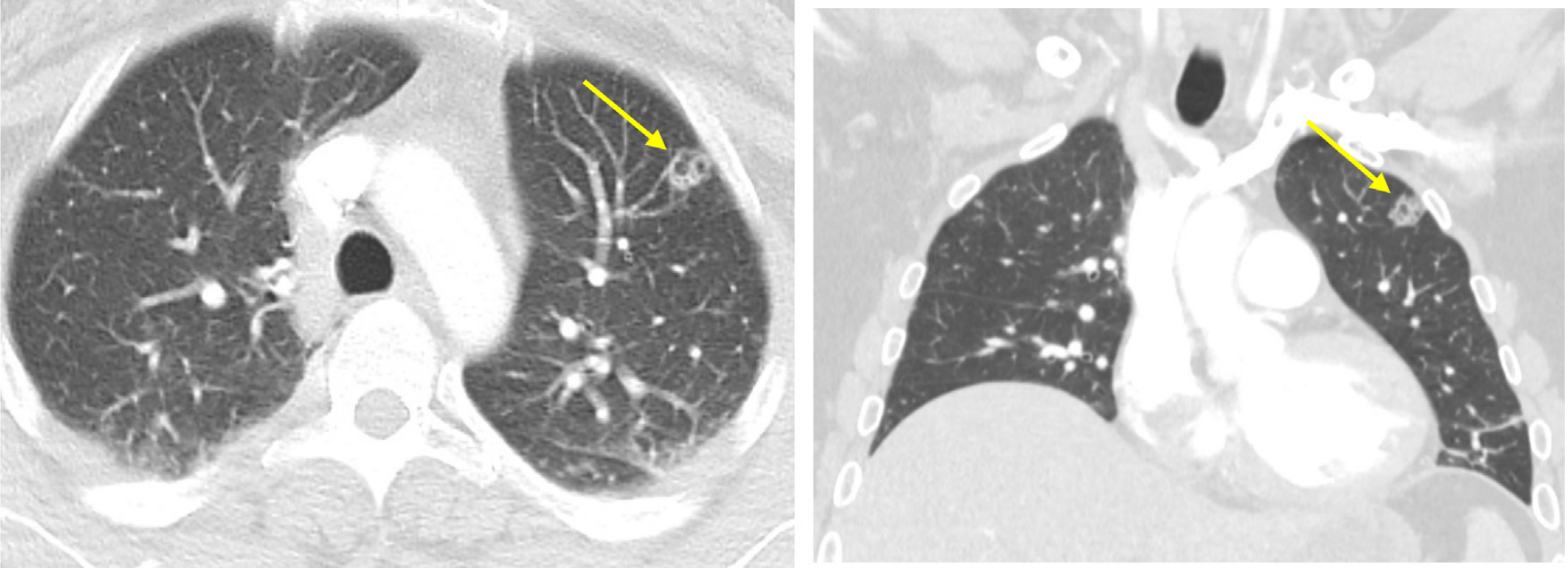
Axial (A) and coronal (B) images of CT chest show multiple bilateral pulmonary nodules, some of which demonstrate central cavitation (yellow arrow).
